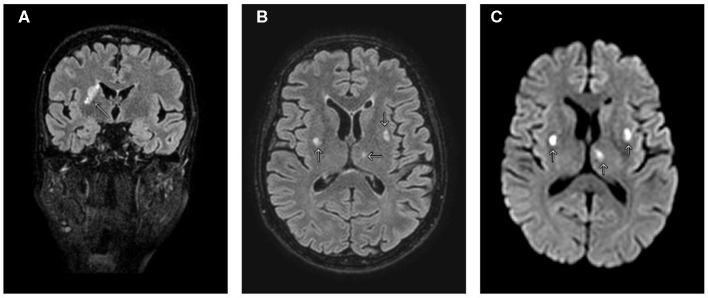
MRI of the brain. (A) T2/fluid-attenuated inversion recovery (FLAIR) coronal view shows hyperintensity in the right caudate, internal capsule, and putamen. (B) T2/FLAIR axial view shows additional lesions involving left thalamus and lenticular nuclei. (C) Diffusion-weighted imaging axial view shows three lesions with restricted diffusion. Arrows indicate CNS lesions.
